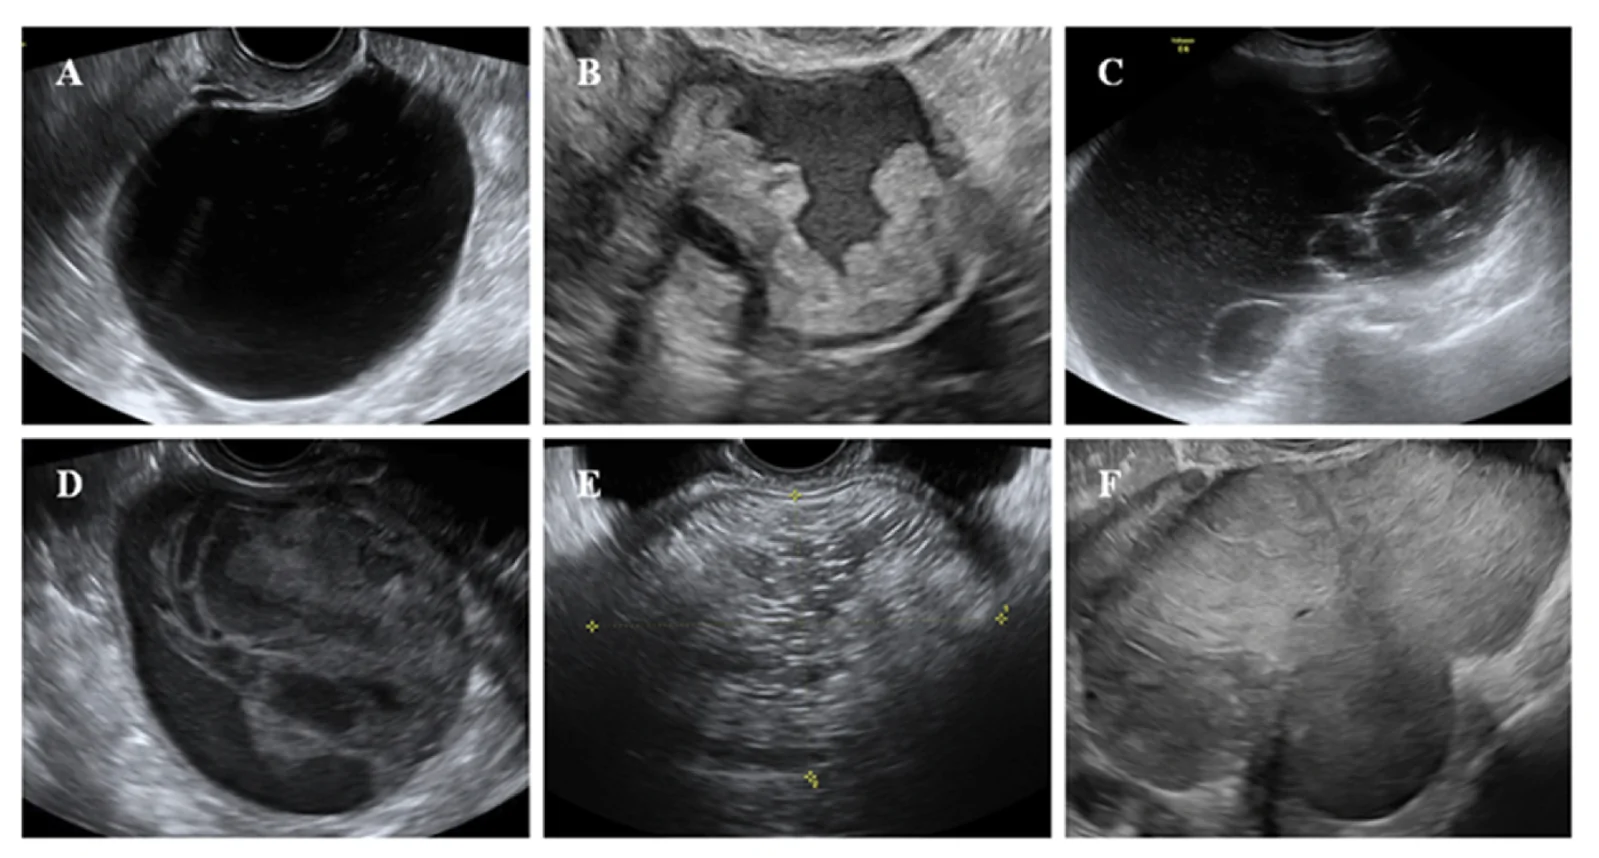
Ultrasound images showing adnexal mass echogenicity, according to IOTA term and definitions: anechoic (A), ground glass (B), low level (C), hemorrhagic (D), mixed (E), not applicable/solid mass (F).
